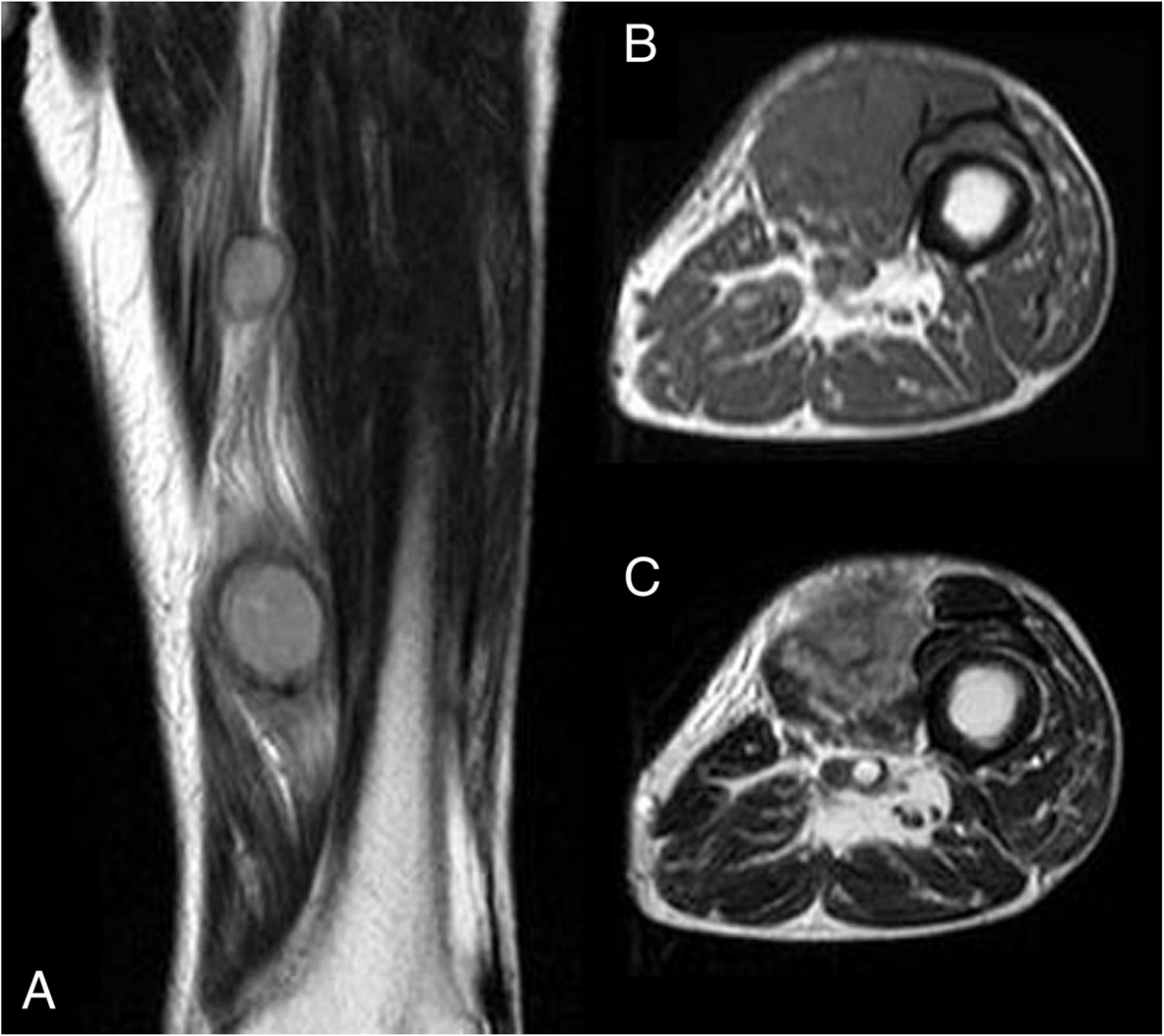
Magnetic resonance imaging of the tumors. (A) A T2-weighted coronal magnetic resonance imaging scan showed two soft-tissue tumors in the intramuscular layer between the vastus medialis muscle and the adductor muscle. Extensive peritumoral inflammatory edema was obvious. (B) A T1-weighted axial magnetic resonance imaging scan of the distal tumor (size, 4.0 × 4.0 × 3.5cm) showed homogeneous low signal intensity. (C) A T2-weighted axial magnetic resonance imaging scan showed heterogeneous low and high combined intensities.
